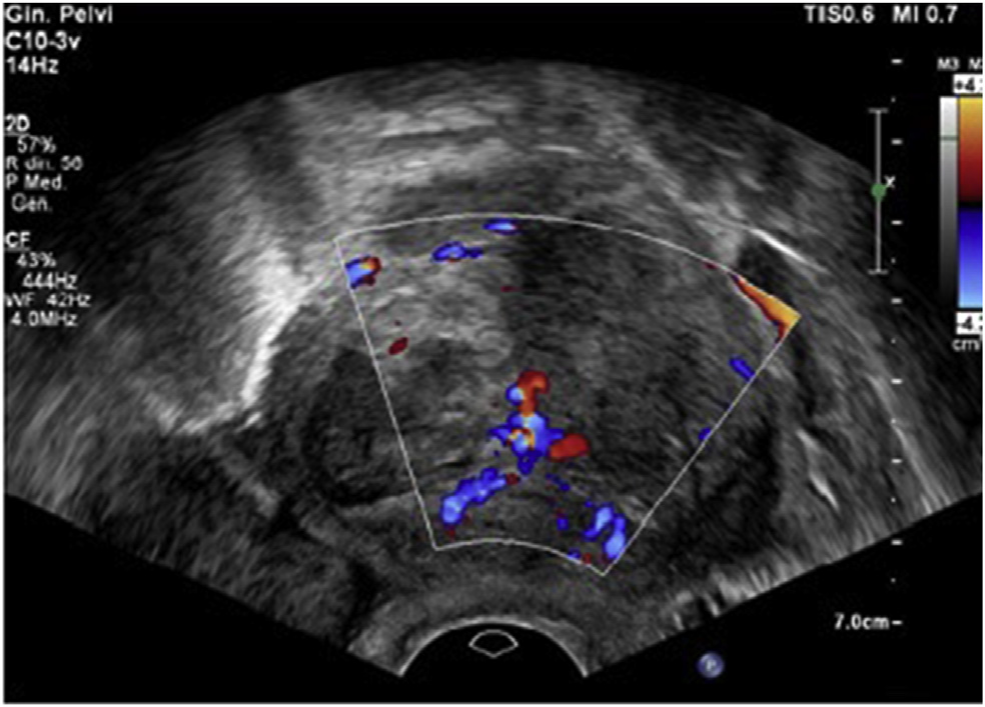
Left ovary ultrasound imaging: irregular dishomogeneous solid mass of 63 × 62 × 60 mm with two hypoechoic cysts and regular margins. Color score 4.
